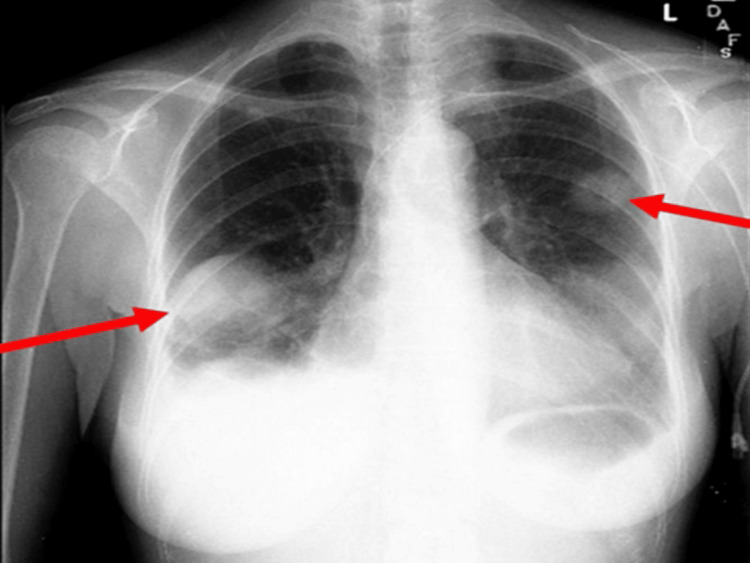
Chest radiograph demonstrating multiple bilateral cavitary pulmonary nodules with surrounding consolidation (arrows).
